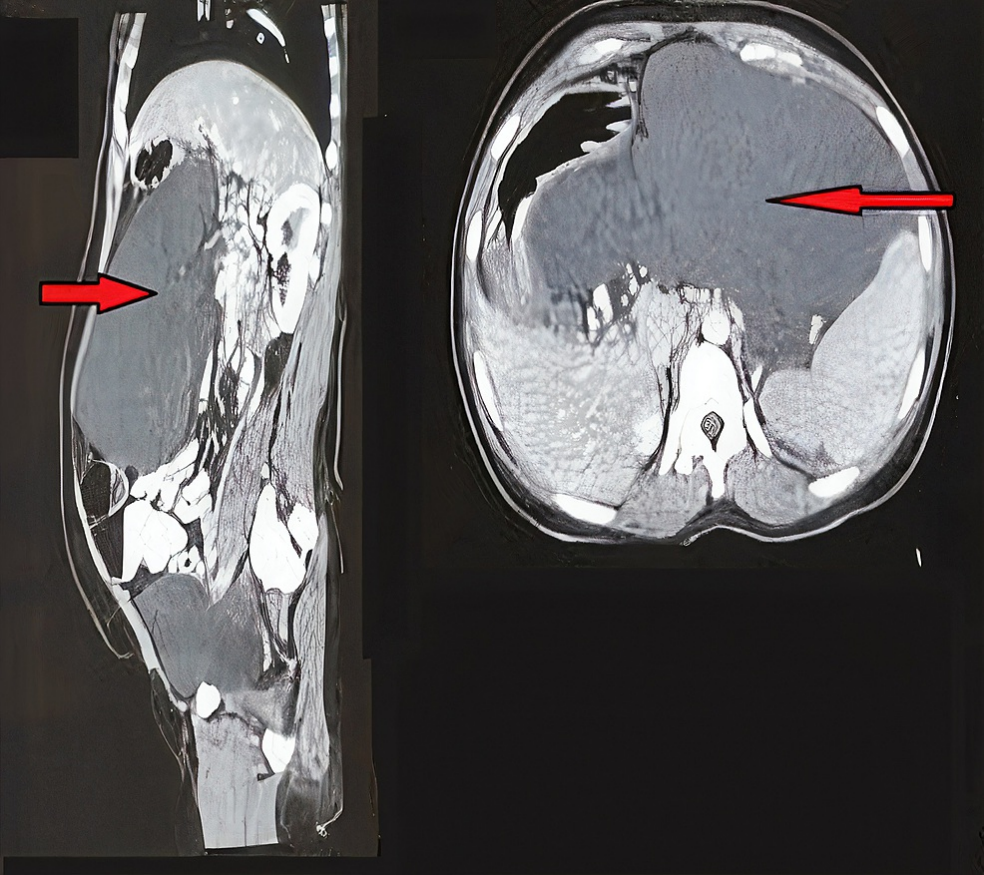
CECT abdomen showed large multiseptated cystic lesion occupying almost the entire abdomen and adhered to pancreas with mass effect. Lesion was well defined, lobulated, and hypodense in nature. CECT, contrast-enhanced computed tomography.
